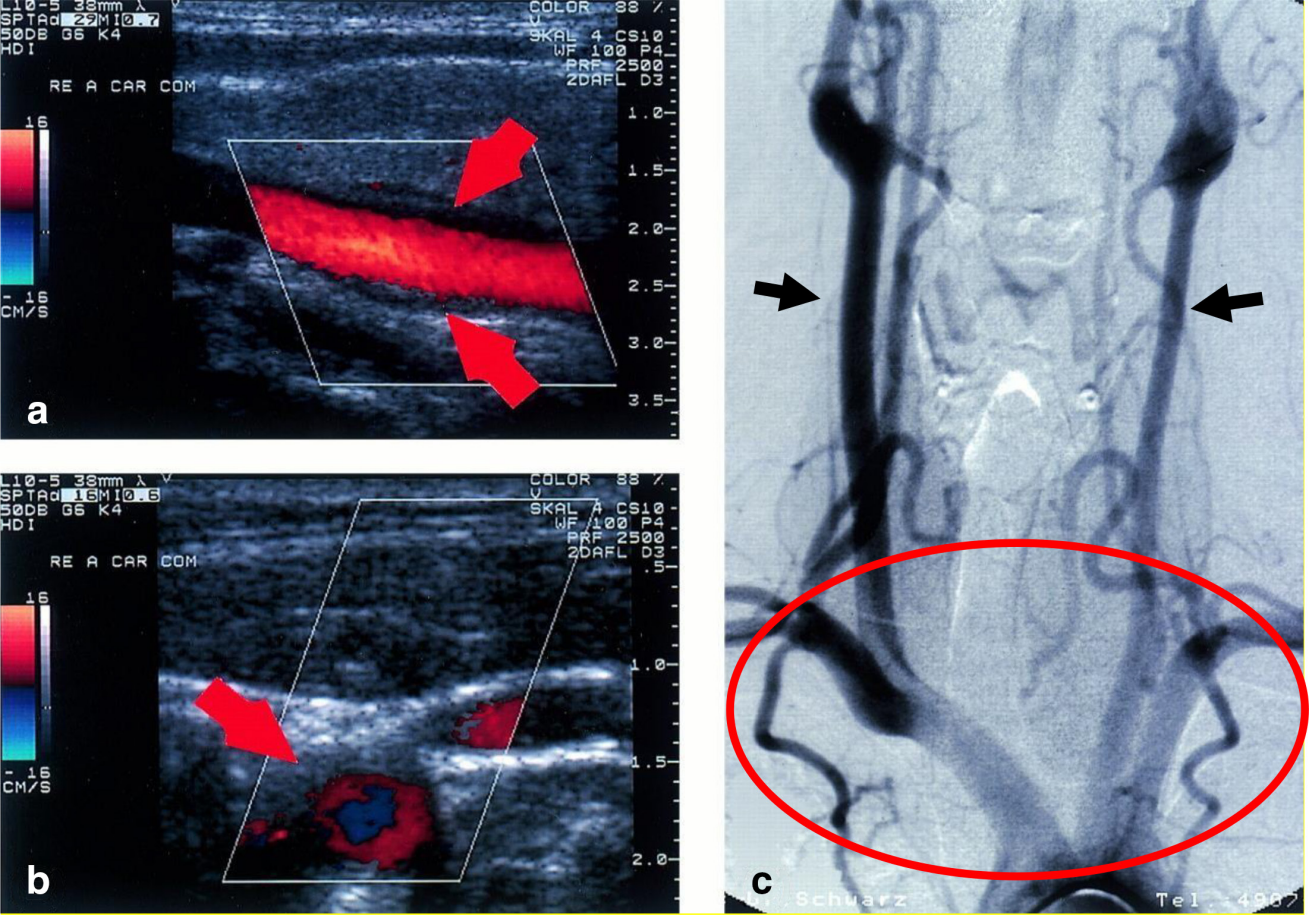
(a) Longitudinal plane view of characteristic ultrasound finding in early TA. (b). Transverse plane view of the same finding. (c). Angiographic confirmation of the diagnosis of TA, revealing 40% stenosis of the right common carotid artery with accompanying post-stenotic dilations well visualized in the right common carotid artery, left proximal common carotid artery, proximal right vertebral artery, and proximal right subclavian artery (circle). Note difference in thickness of right and left common carotid arteries (arrows). Reproduced from Schmidt, W.A,; Nerenheim, A., Diagnosis of early Takayasu arteritis with sonography, Rheumatology, 2002, 41, five by permission of Oxford University Press. TA, Takayasu arteritis.
